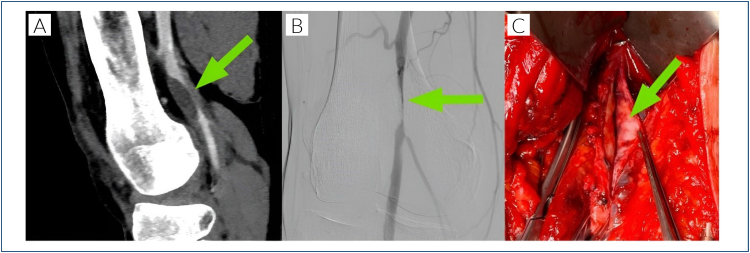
(A) A computed tomographic image showing the location of the stenosis at the P1 segment of the popliteal artery. (B) A digital subtraction angiography showing the eccentric, gradually rising stenosis. (C) An intraoperative image exhibiting the opened adventitial cyst with all gelatinous contents removed.
